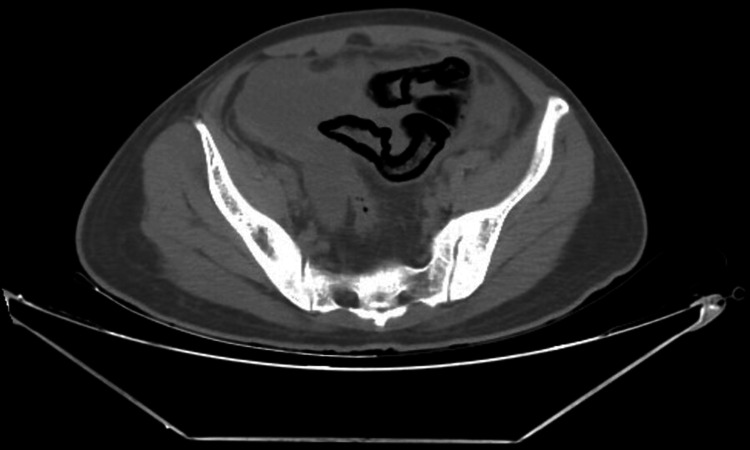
CECT abdomen axial view showing pneumatosis intestinalis CECT: contrast-enhanced computed tomography
